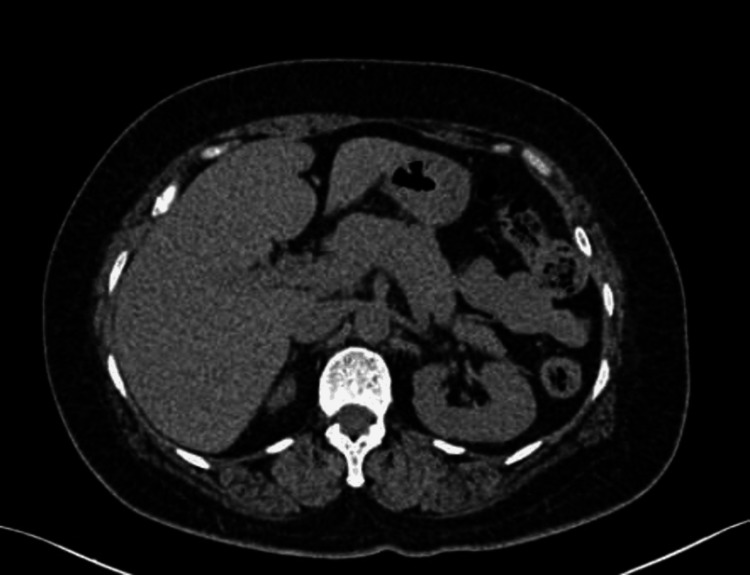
Normal abdominal computed tomography (CT)
